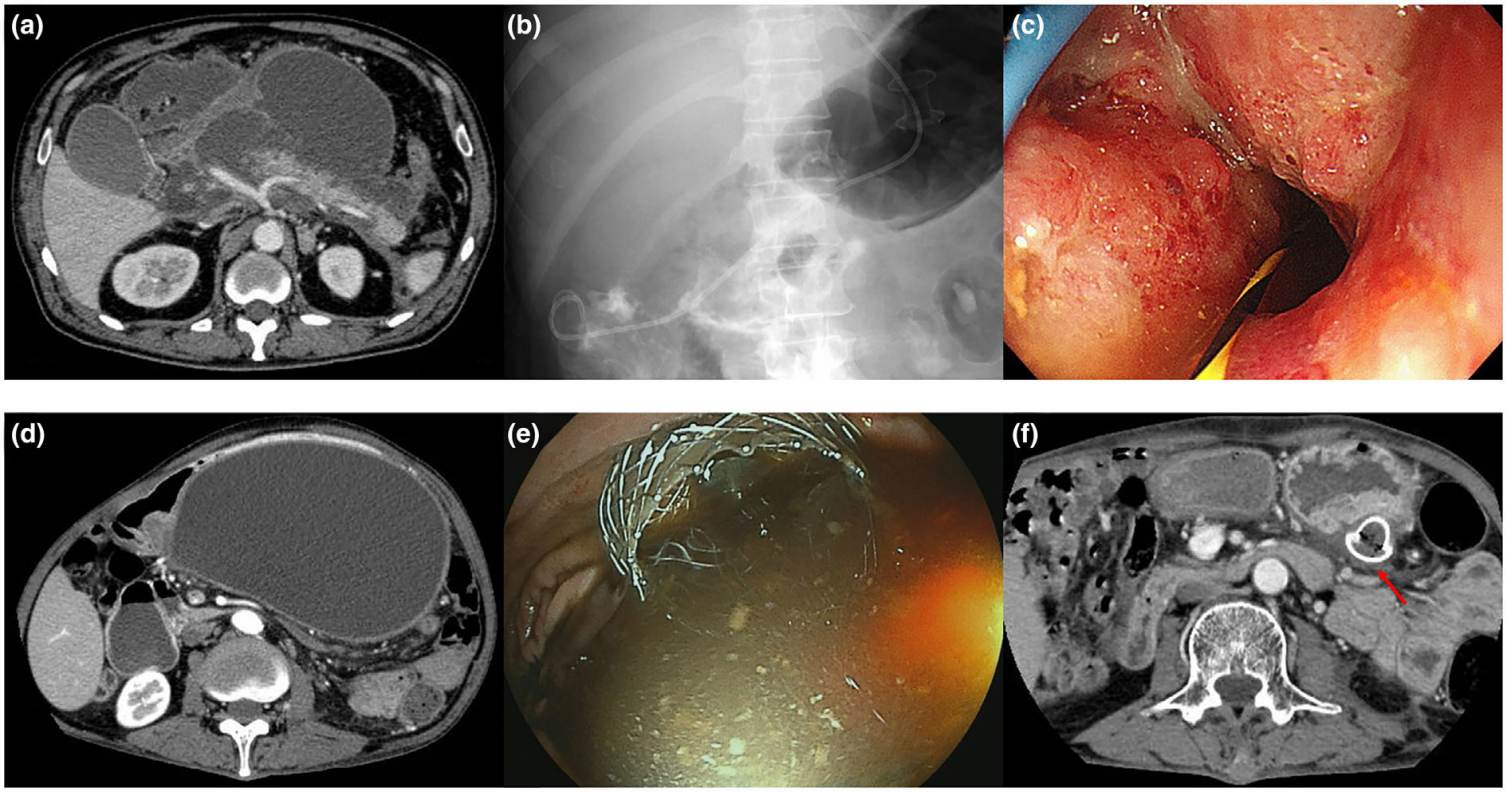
Procedures of endoscopic ultrasound (EUS)‐guided treatment of pancreatic fluid collections in a clinical multi‐institutional cohort within the WONDERFUL consortium (a–c, walled‐off necrosis [WON]; d–f, pseudocyst). (a) Contrast‐enhanced computed tomography delineating large WON extending to the pelvic cavity. (b) Fluoroscopic image of EUS‐guided drainage via the transgastric placement of a lumen‐apposing metal stent (LAMS) and coaxial nasocystic catheter. (c) Endoscopic image showing successful clearance of necrotic contents after four sessions of endoscopic necrosectomy. (d) Contrast‐enhanced computed tomography delineating a large pseudocyst. (e) Endoscopic image after EUS‐guided transgastric placement of a LAMS with a discharge of infectious intracystic fluid. (f) Contrast‐enhanced computed tomography delineating clinical treatment success with a reduction in the size of the pseudocyst (arrow, a LAMS in situ).
